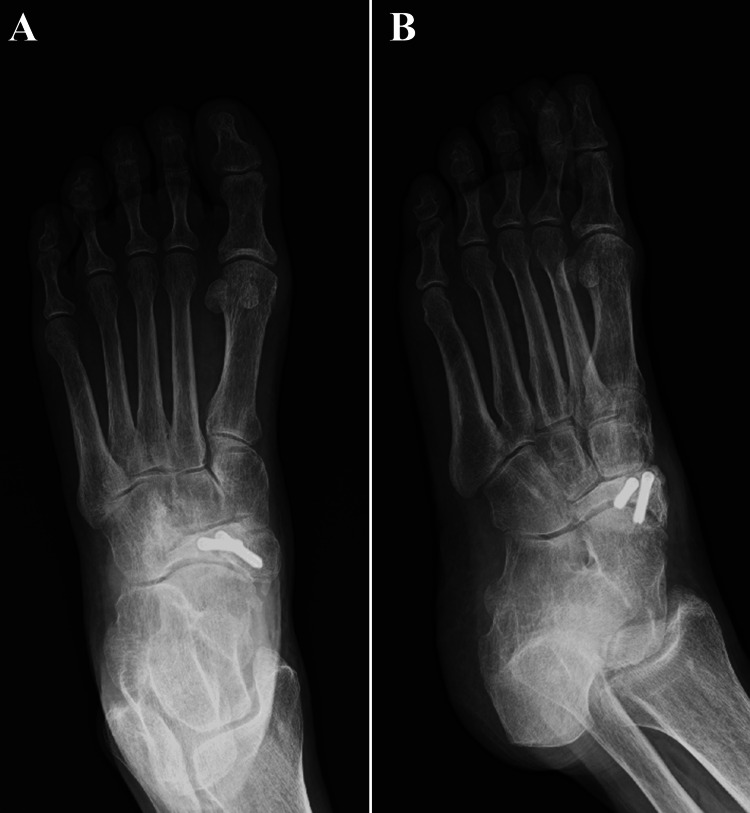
Postoperative plain radiographic findings. (A) Anteroposterior view and (B) internal oblique view.
